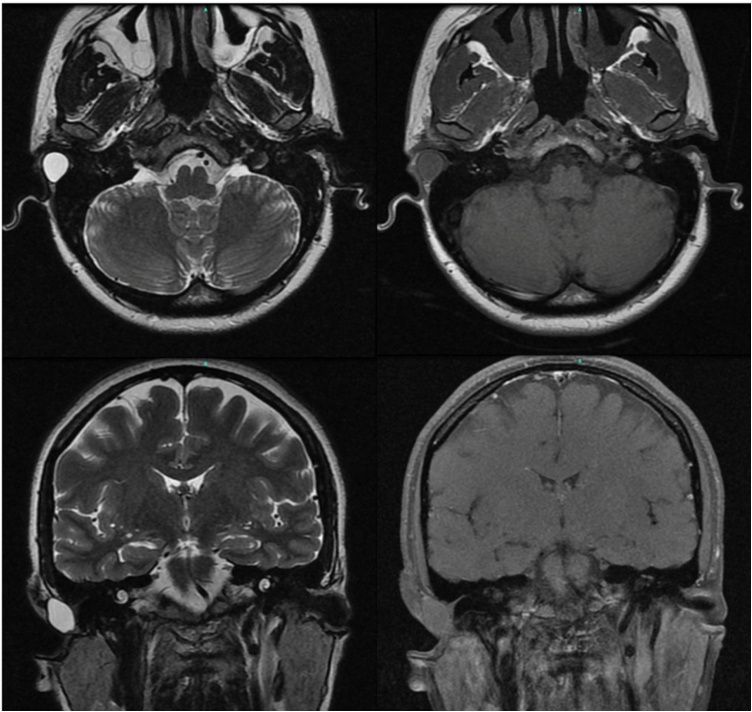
MRI shows a cystic lesion in the posterior-superior right external auditory canal, isointense on T1, hyperintense on T2, with no gadolinium enhancement or diffusion restriction.
